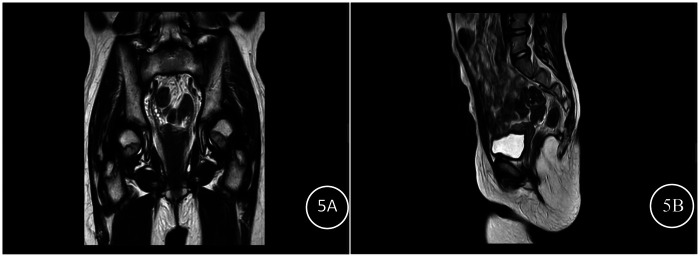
Pediatric MRI findings (A) pelvic MRI coronary view; (B) pelvic MRI T2 sagittal view.
